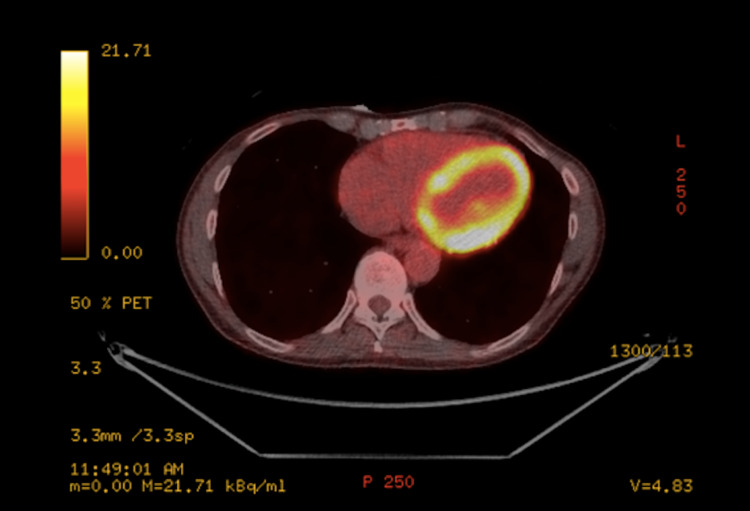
18F-FDG PET at baseline demonstrates increased uptake in the lateral and inferior walls of the left ventricle 18F-FDG PET: 18F-fluorodeoxyglucose positron emission tomography
